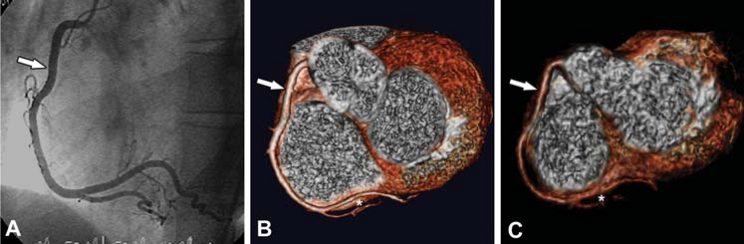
Angio (*A*) and noninvasive coronary angiography using MSCT (*B*) and MRI (*C*) all demonstrate absence of significant stenoses in the right coronary artery (arrow) in a 45-year-old female patient with atypical angina pectoris. Note that MSCT due to higher spatial resolution allows better delineation of the distal segments of the right coronary artery than MRI (asterisks).
